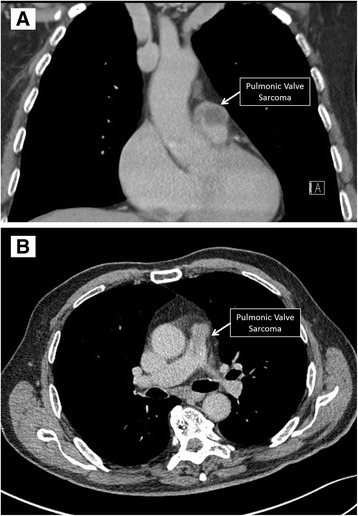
CT. Contrast-enhanced chest CT demonstrates spindle-cell sarcoma at the level of the pulmonic valve on a coronal (Panel a) and axial cut (Panel b)
